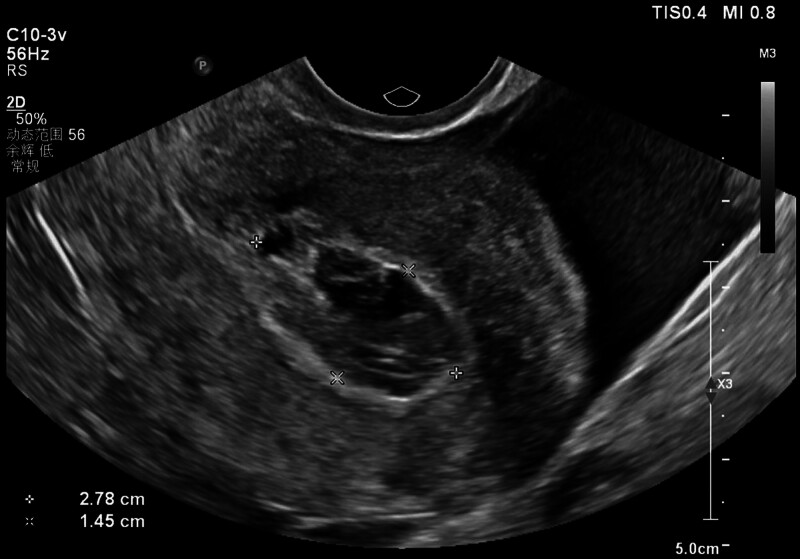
Transvaginal ultrasonography revealed a heterogeneous hyperechoic intrauterine fluid collection (2.78 × 1.45 cm).
